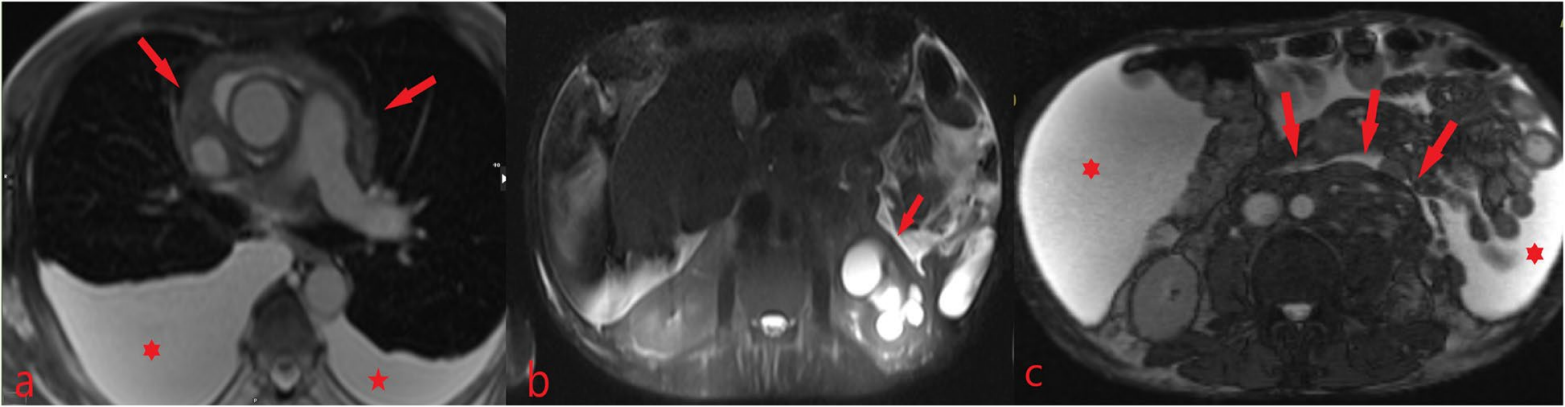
Magnetic resonance imaging shows 6a: bilateral pleural effusion and mediastinal infiltration involving vascular structures,6b: hydronephrosis and atrophic changes in the left kidney and 6c: ascites and retroperitoneal infiltration
